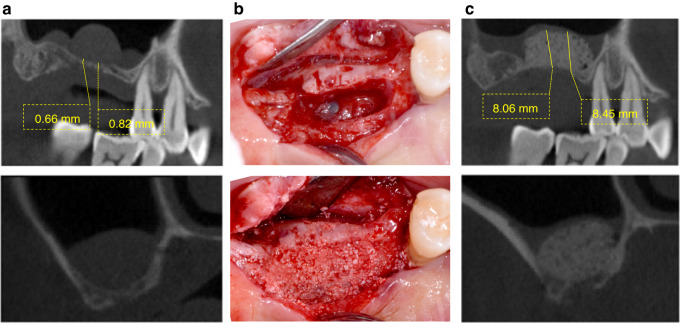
Case 1: a Pre-operative CBCT assessment, b surgical procedure, and c post-operative CBCT assessment of patient in case 1
